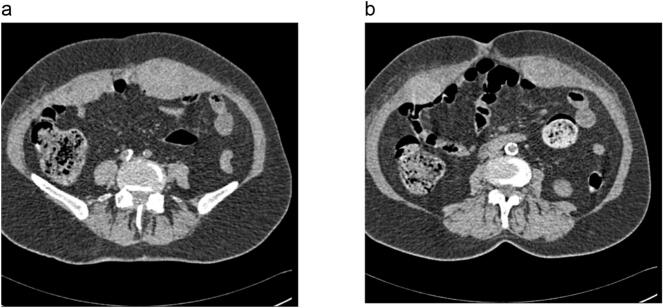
(a, b). CT of the abdomen with a Hematoma of the right and left rectus abdominis muscles.
